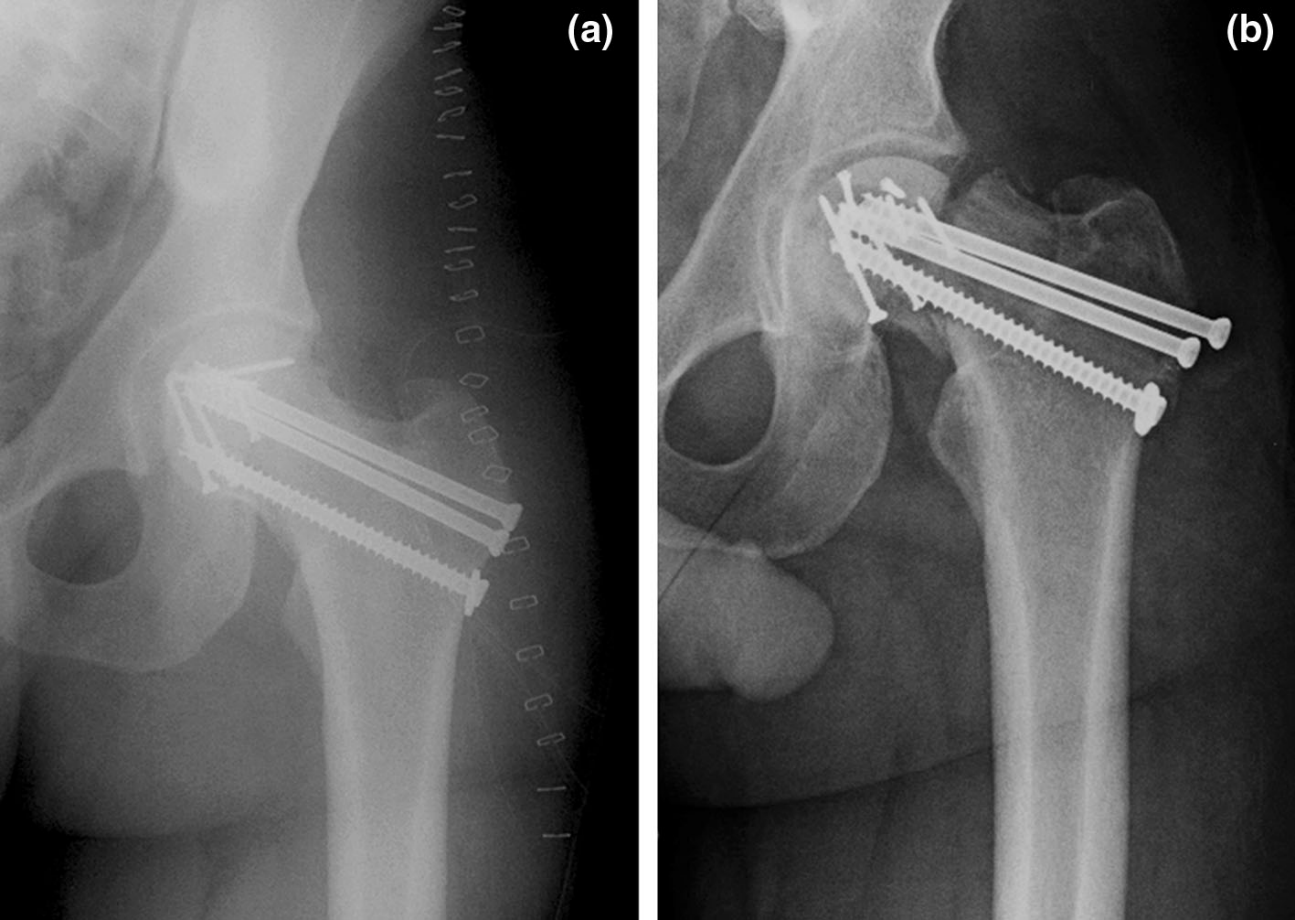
a Postoperative AP radiograph of a 28-year-old male who sustained a gunshot wound to the left hip and presented with a Pipkin III fracture of the femoral neck and head. Minifragment screws were used for femoral head fixation; cannulated screws were used for femoral neck fixation. b Three-month follow-up AP radiograph of the patient shown in a. Radiographs show catastrophic failure of fixation that required conversion to a total hip arthroplasty
